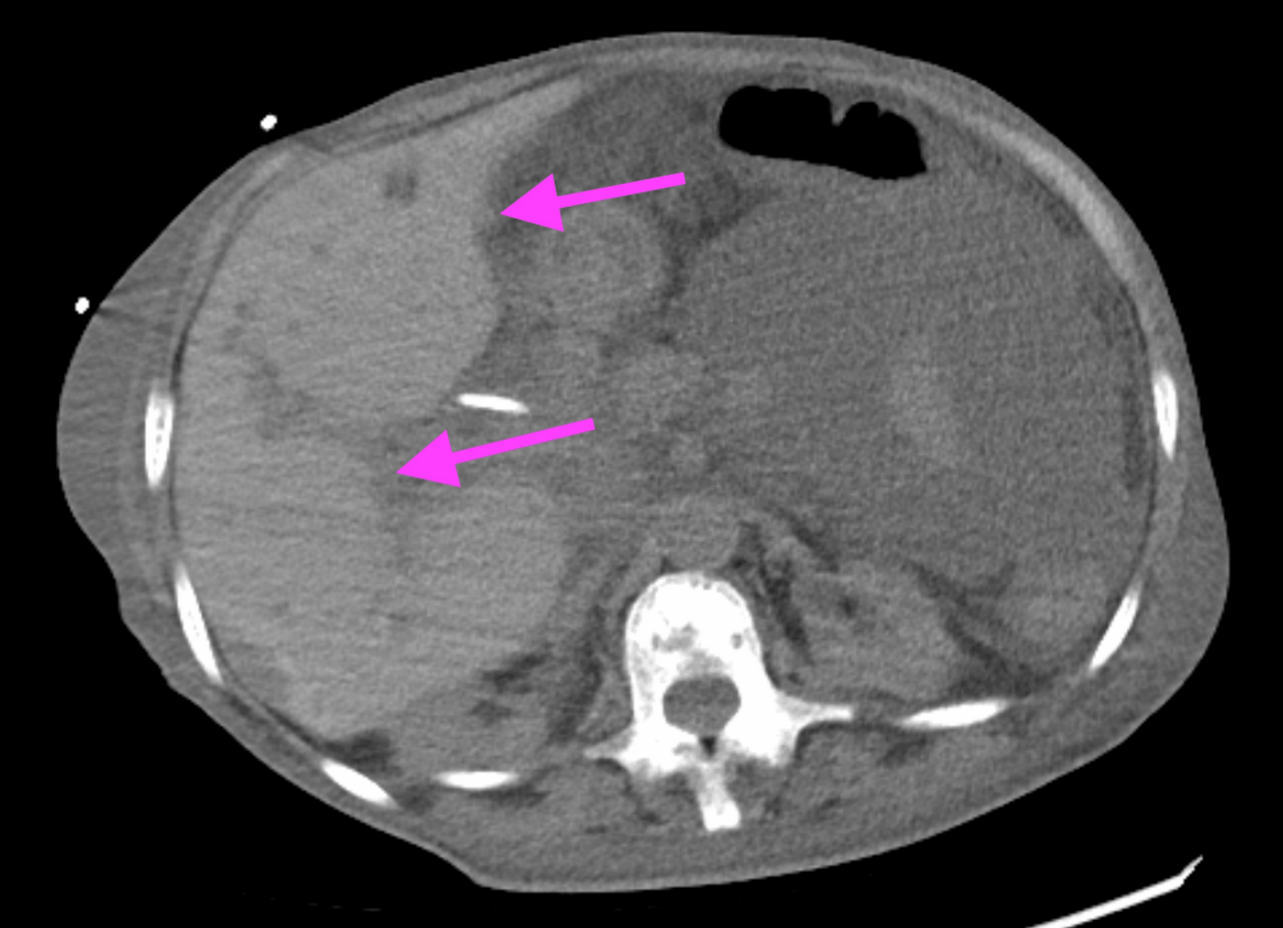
The final CT of the abdomen and pelvis showed a scalloping contour of the liver, possibly a peritoneal mass, cholelithiasis, and a large amount of ascites with ascending colon wall thickening, suggesting colitis, with arrows highlighting the areas of scalloping.
